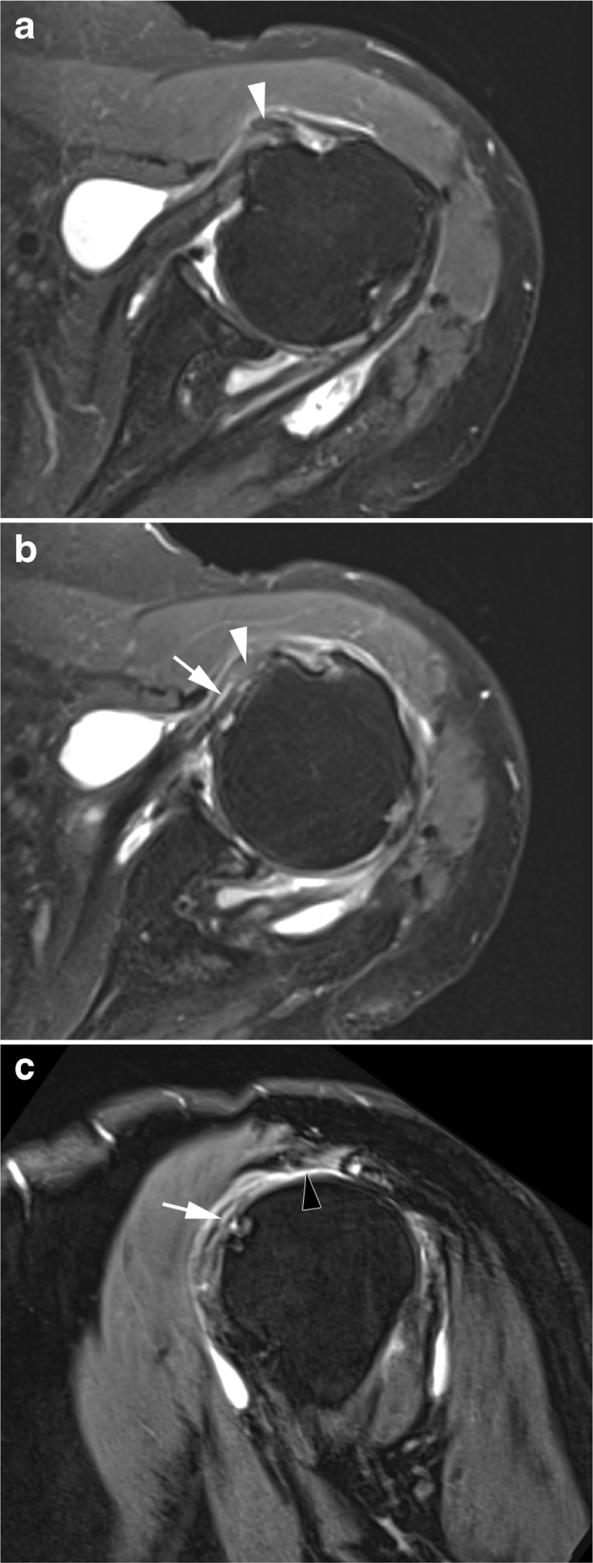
Left shoulder MRI of a 76-year-old woman. On axial FS PD-weighted images, the LHBT (white arrow heads) is medially displaced (a), and incarcerated into a cleavage of the SSC tendon (white arrow (b). On the sagittal oblique FS T2-weighted image (c), the SSC tendon tear (white arrow) is associated with a complete full thickness tear of the SSP tendon (black arrow head)
